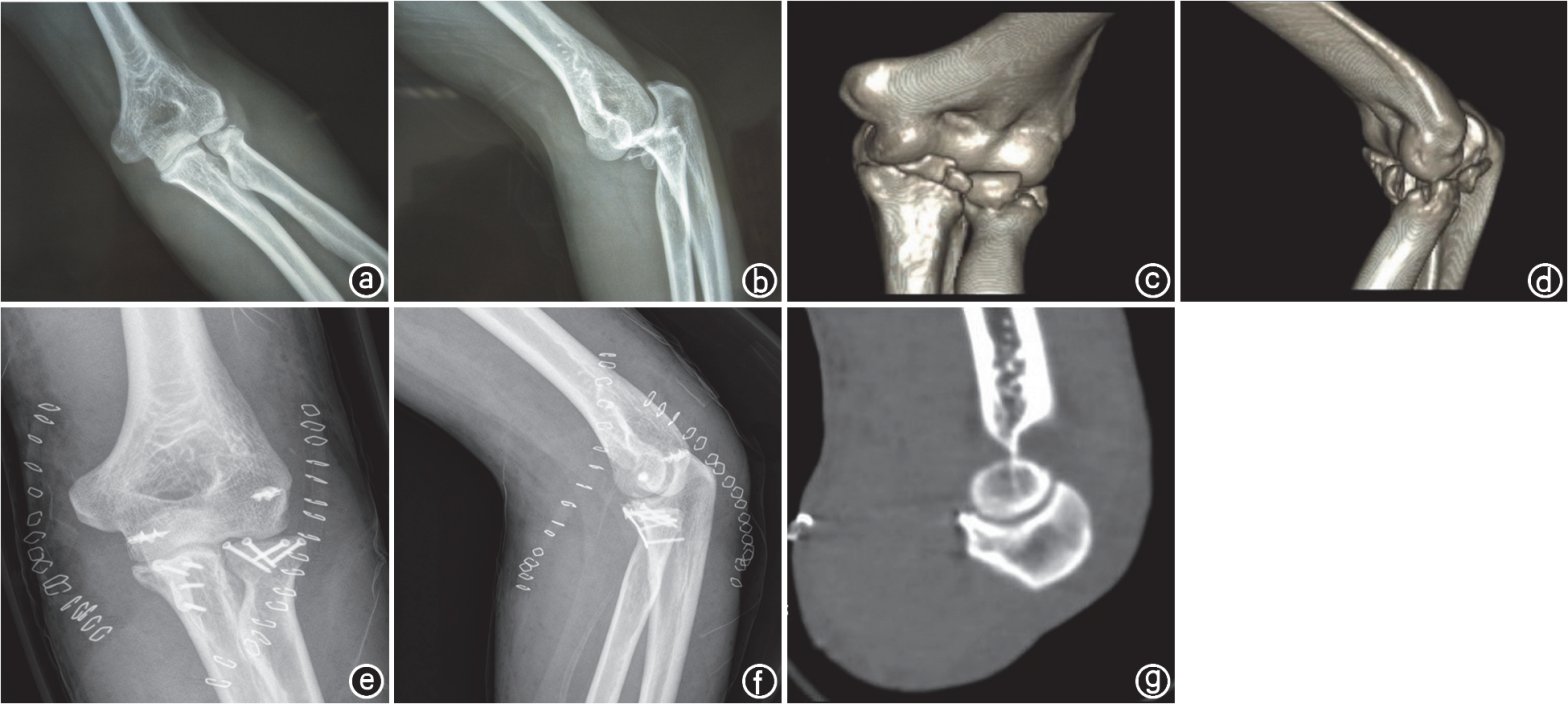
The male patient aged 47 years was admitted 2 days after left elbow injury induced by falls. a,b. Anteroposterior and lateral X-ray films before operation shows dislocation of left elbow joint, ulnar coronoid process fractures, and radial head fractures; c,d. Three-dimensional CT reconstruction before operation shows dislocation of left elbow joint, comminuted fractures of ulnar coronoid process, and comminuted fractures of radial head; e,f. Anteroposterior and lateral X-ray films were taken after fixation was conducted by applying medial and lateral elbow approaches; g. Three-dimensional CT reconstruction of vertical plane after operation indicates that elbow joint recovers with concentric reduction.
